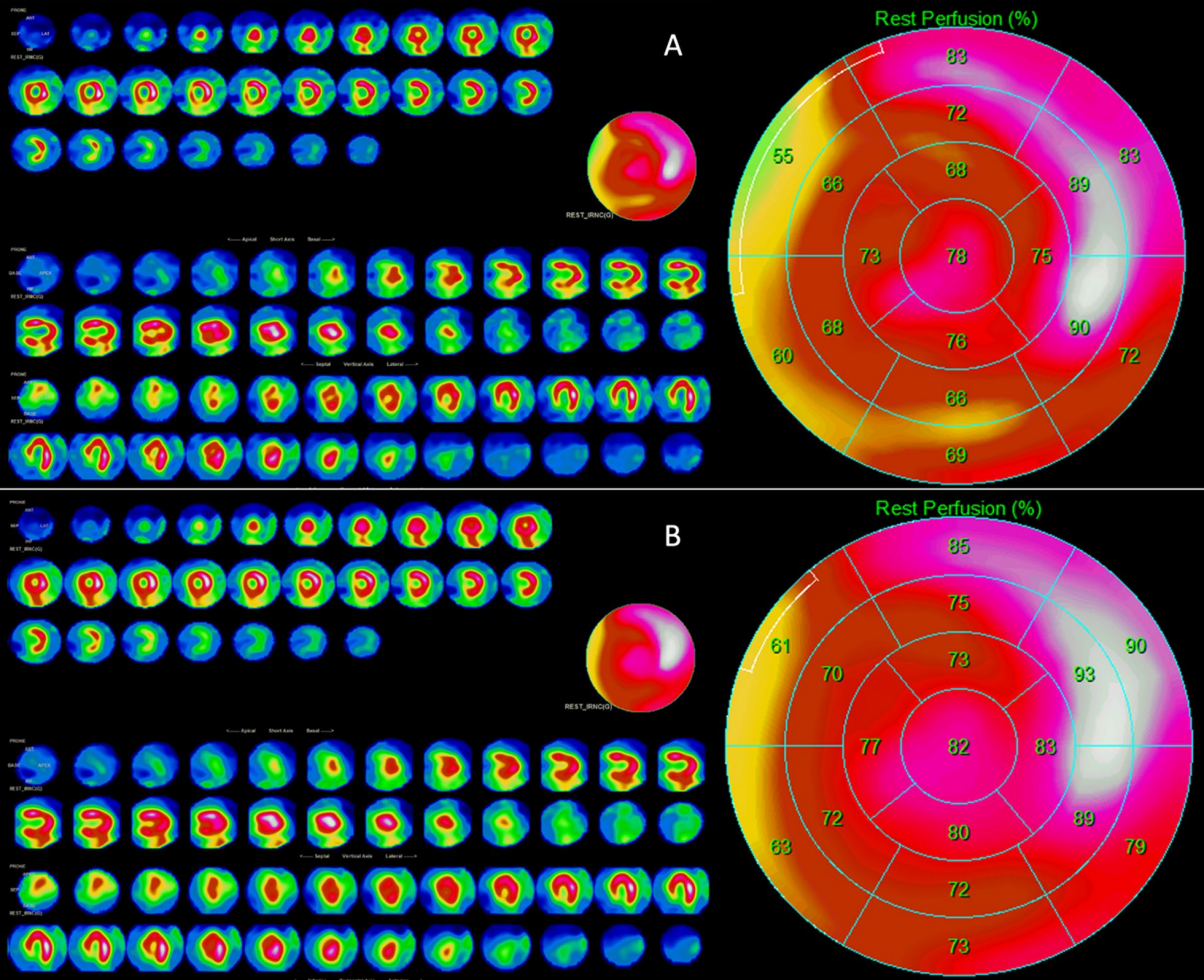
Representative images of reconstructions A and B on the same scintigraphy acquisition. Summed stress or rest score without septum after reconstruction A (SSRSwsa) = 5; Summed stress or rest score without septum after reconstruction B (SSRSwsb) = 0; ∆ws = 5; ∆rws = 100%
